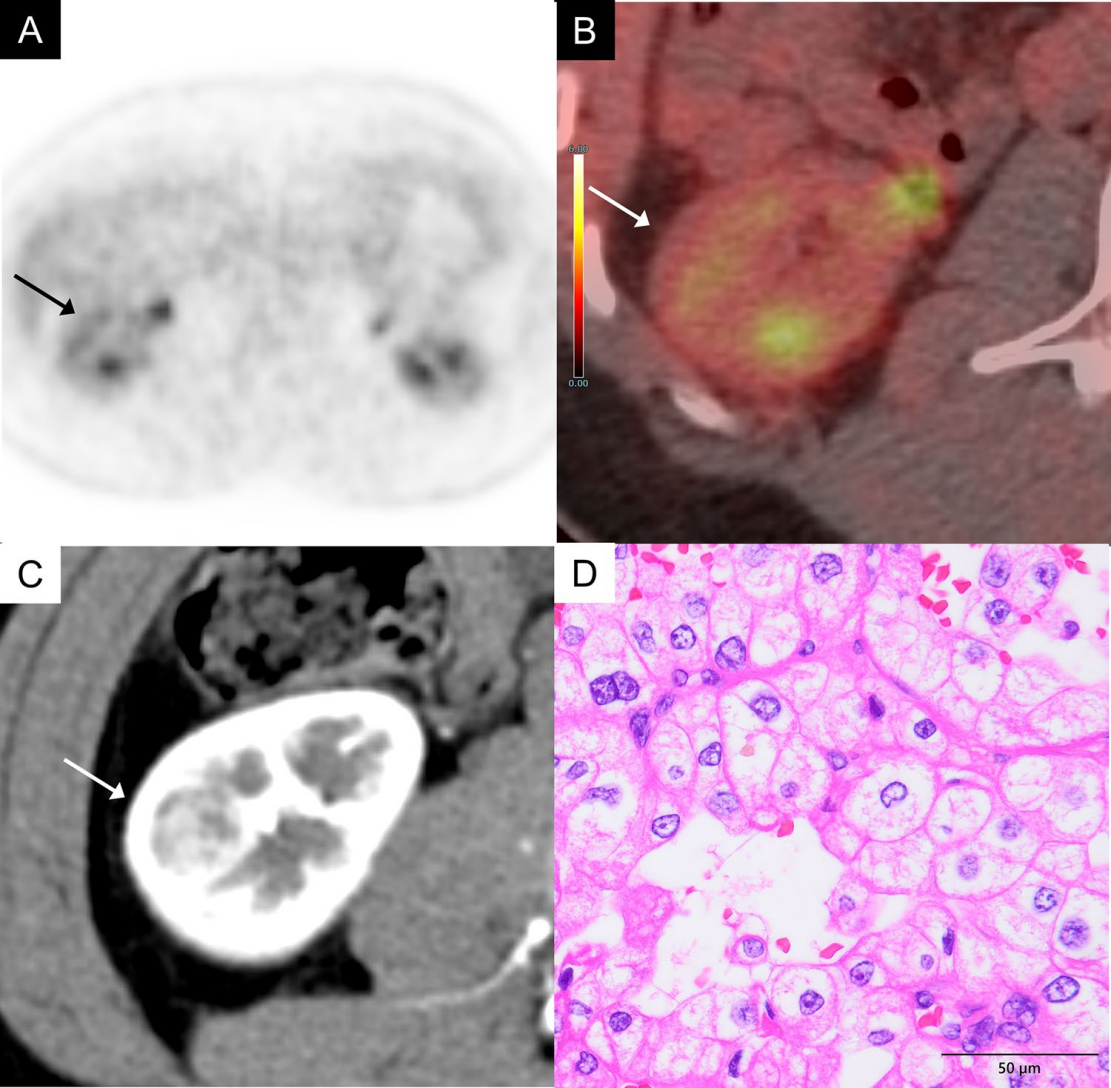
A 35-year-old man with a chromophobe renal cell carcinoma (WHO/ISUP grade 3) of the right kidney. A In the FDG-PET image (grayscale), the uptake of the left renal tumor (arrow) is almost the same as that of the renal cortex, making tumor identification difficult. B Axial FDG-PET/CT image shows mild FDG uptake in a mass of the right kidney (arrow), showing an SUVmax 2.7, while normal renal cortex shows an SUVmax 2.1. C Axial contrast-enhanced CT in the corticomedullary phase shows a heterogeneous enhancement in the mass (arrow). D The tumor cells have wrinkling nuclear membranes with eosinophilic cytoplasm. Some tumor cells have perinuclear halo or binuclear
